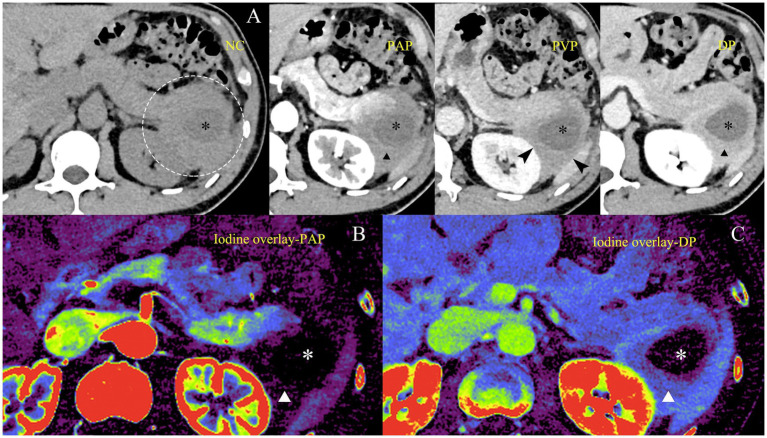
Multiphasic CT and iodine map images of the pancreatic lesion. (A) Axial contrast-enhanced CT demonstrates a cystic-solid lesion infiltrating the pancreatic tail (dotted circle). The cystic component (black asterisk) shows no enhancement, while the solid portion (black triangle) exhibits mild enhancement on the pancreatic parenchymal phase (PAP) and progressive enhancement on the delayed phase (DP). The lesion abuts the left kidney and spleen [black arrowheads, portal venous phase (PVP)] with loss of the intervening fat plane but without definite invasion. No typical IgG4-related changes are observed in the kidneys, aorta, or other abdominal organs. (B,C) Iodine maps on PAP and DP show mild iodine uptake in the solid component (white triangle) and absence of measurable iodine in the cystic portion (white asterisk). The gradual increase in iodine concentration in the solid component during DP parallels its progressive enhancement, suggesting fibrous stroma or low vascularity. NC, non-contrast; PAP, pancreatic parenchymal phase; PVP, portal venous phase; DP, delayed phase.
